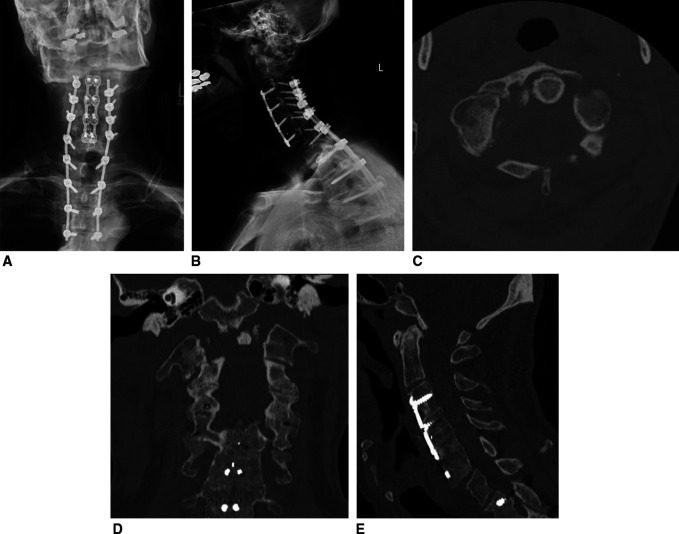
Radiograph demonstrating A, AP and (B) Lateral x-rays of the patient's cervical spine demonstrating a displaced and comminuted C1 burst fracture with nonunion. Previous C3 to C6 ACDF done a few years ago and C3-T4 posterior cervical fusion done 6 months earlier for a C6 facet fracture also shown. C, Axial (D) coronal, and (E) sagittal CT images of the patient's fracture, demonstrating right-sided displacement of C1 with cranial settling and posterior displacement of the occiput with fracture nonunion of C1. ACDF = anterior cervical discectomy and fusion
